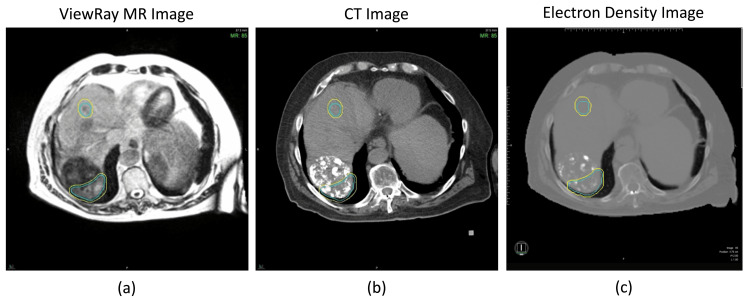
MRI image acquired on 0.35 T ViewRay MRIdian (a) and CT image acquired on Siemens SOMATOM Edge (b) for use in radiation therapy treatment planning of two liver lesions. The corresponding electron density (c) is used for the radiation dose calculation. The blue volumes indicate the gross tumor volumes (GTVs), while the yellow indicates a 3 mm expansion to form the planning target volume (PTV). Note the appearance of a highly attenuating (bright) material in the CT image.
